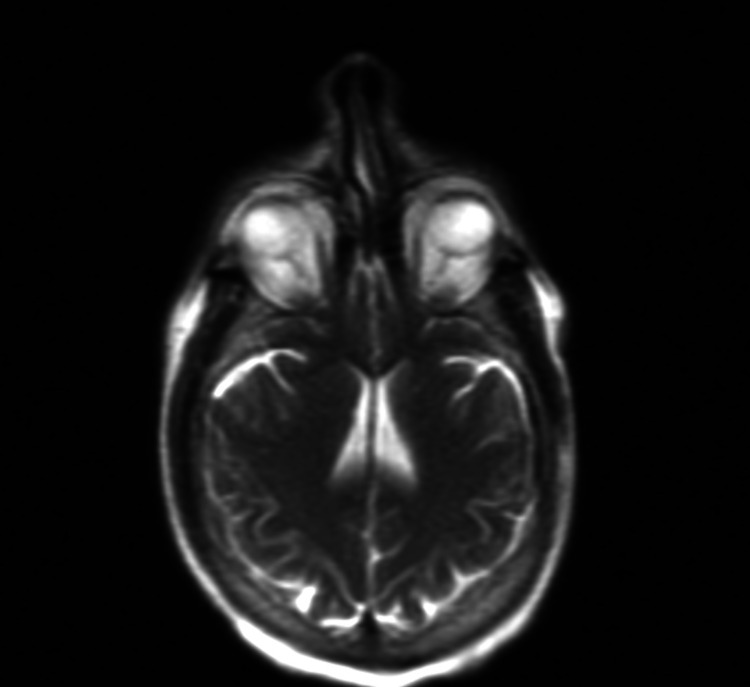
Axial plane of brain MRI with contrast MRI, magnetic resonance imaging.
